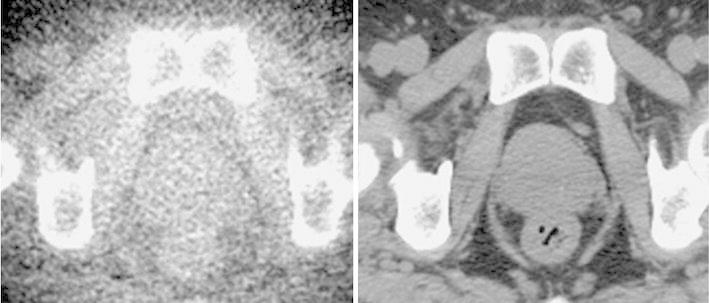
A sample of MV-CBCT image (*left*) and the corresponding kilovoltage CT image at the treatment planning (*right*)
